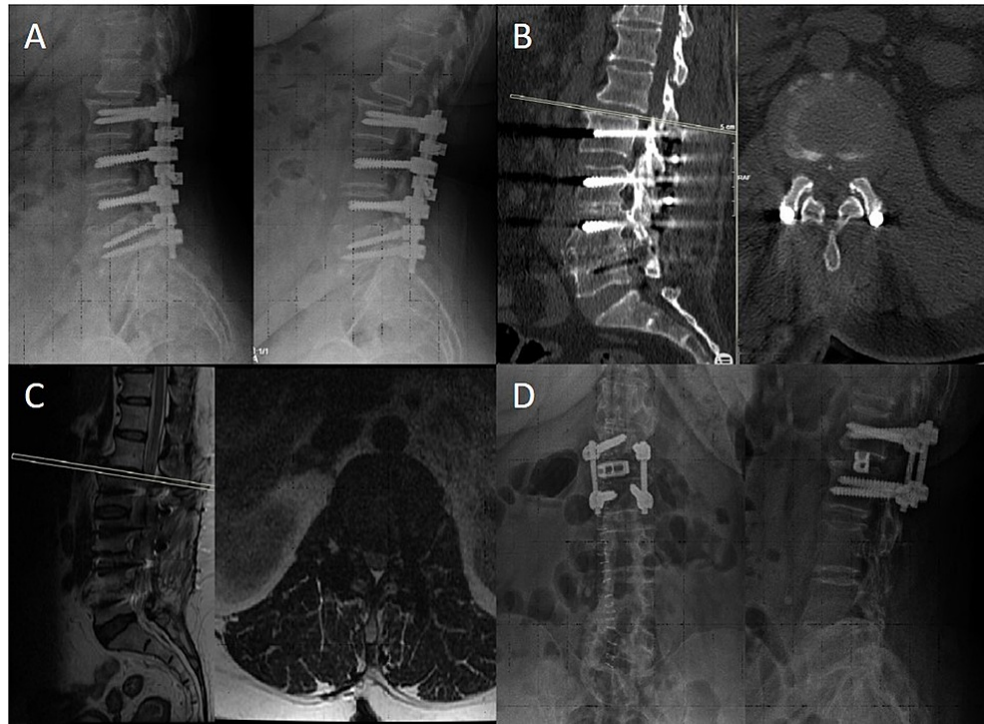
A) Preoperative Dynamic X-ray Images, B) Preoperative CT, C) Preoperative MRI, D) Postoperative Two-Way X-ray Images A-C: In the preoperative radiological images, it is seen that the adjacent segment, L1-L2 instability, and disc herniation developed in the patient who underwent L2-L3-L4-L5 posterior transpedicular stabilization. D: Since there was fusion in the lower segments, the transpeduncular screws in the lower segment were removed. Transpedicular stabilization of the L1-L2 level was performed with transforaminal interbody cage fusion. It shows the postoperative control X-ray.
